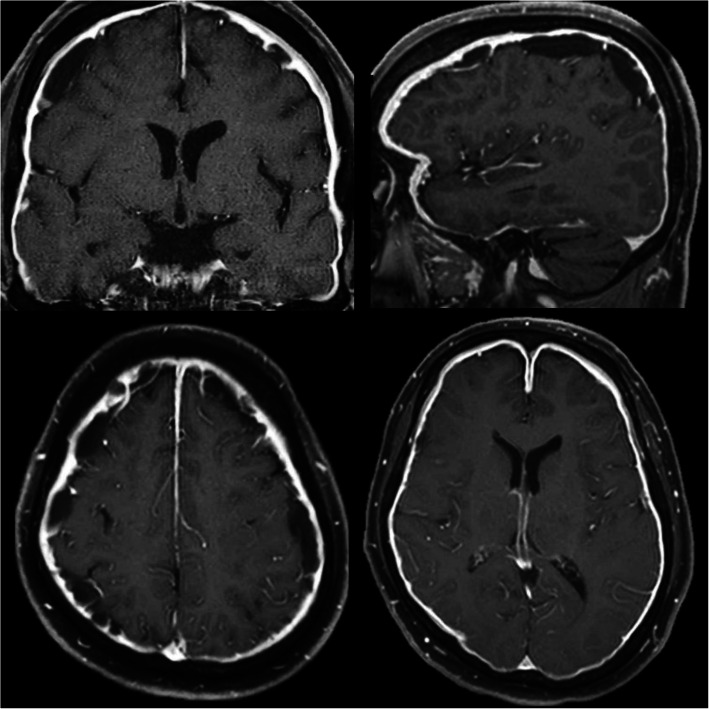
Brain MRI (gadolinium enhanced T1-wighted images) on admission. MRI revealed polycystic subdural hygroma, hematoma, and diffuse thickening of the dura mater when probed using contrast enhancement (MRI, magnetic resonance imaging)
